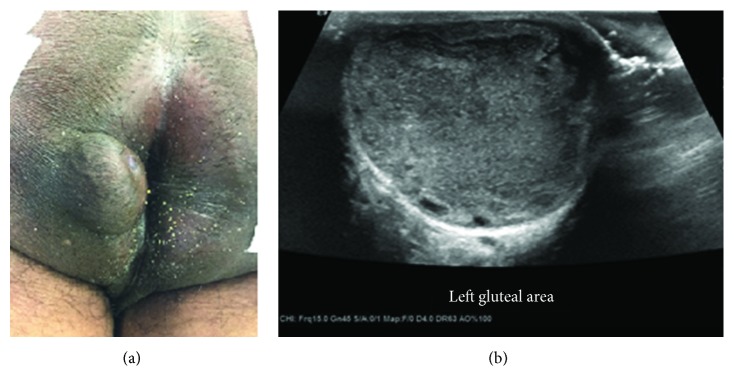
(a) Appearance of the mass at initial presentation. (b) Ultrasound revealed a hyperechoic, well-circumscribed mass.
